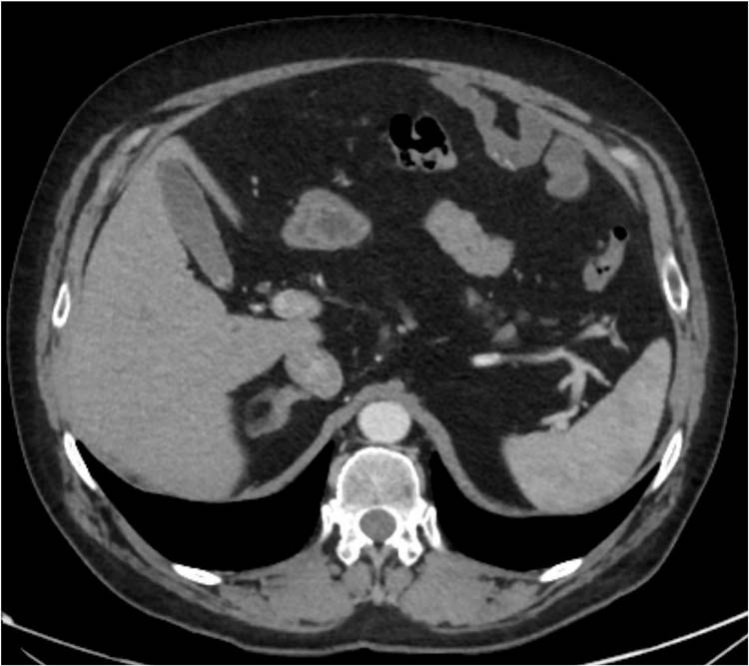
Follow up CT angiogram showing improvement in perihepatic haematoma. Figure 5 is another axial section from the patient’s CT angiogram performed 4 weeks following discharge and shows resolution of the perihepatic haematoma seen previously. Together with the other sections of the CT angiogram and the patient’s overall clinical state, the image represents a significant improvement in the patient’s intra-abdominal bleeding.
